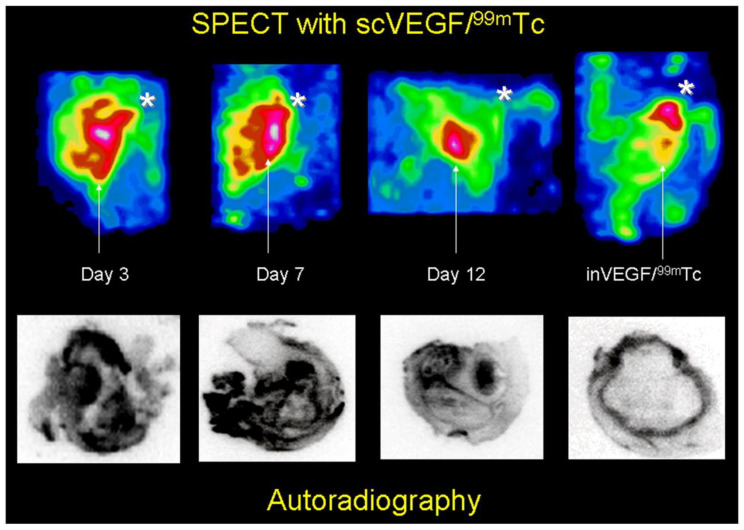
[^99m^Tc]Tc scVEGF SPECT of sterile right thigh abscess. Reprinted from open access source (CC BY 4.0) [130].
